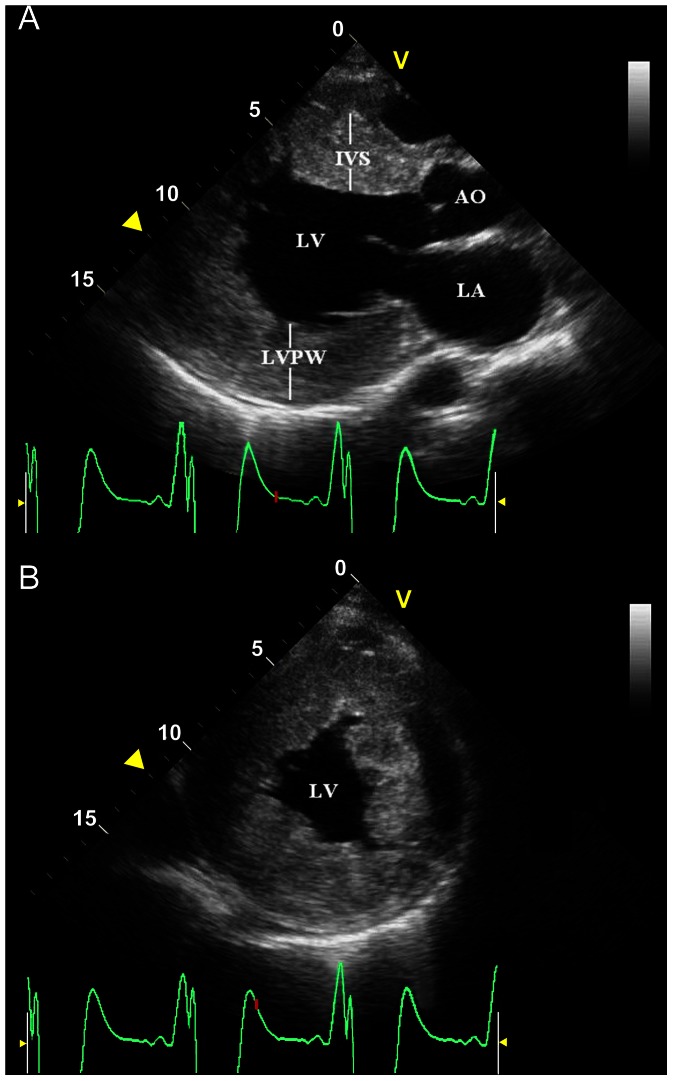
The parasternal long-axis view (A) and short-axis view (B) of an echocardiogram of the proband. It demonstrates severe concentric left ventricular (LV) hypertrophy with enlarged left chambers. AO, aorta; IVS, interventricular septum; LA, left atrium; LVPW: left ventricular posterior wall.
